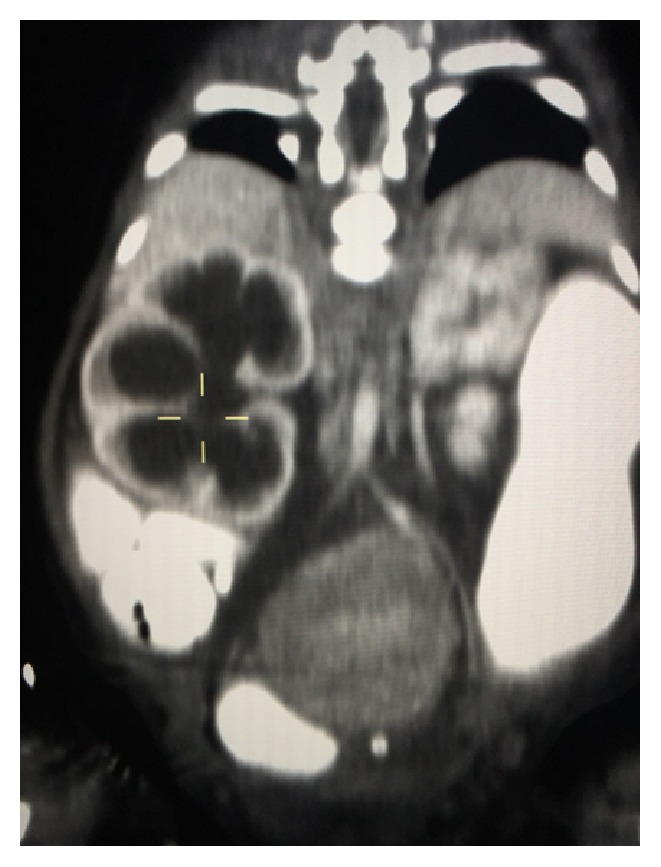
CT image showing mass on presacral space and hydroureteronephrosis.
